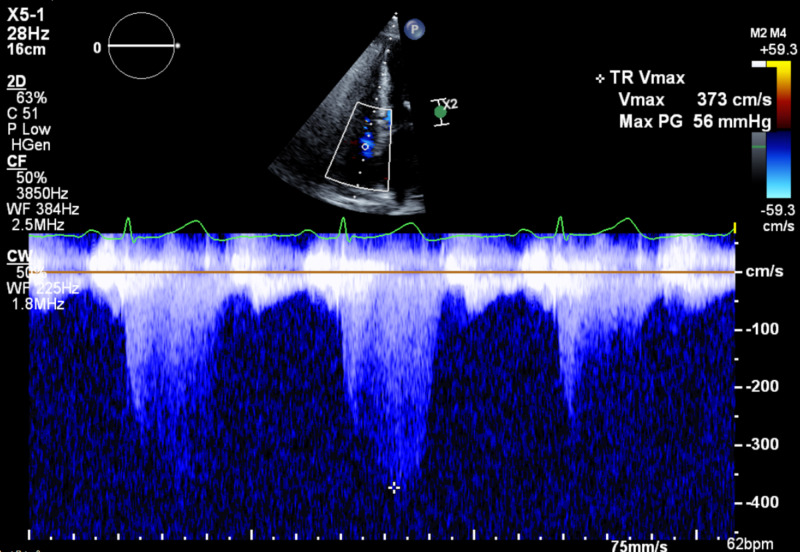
Initial TTE showing tricuspid regurgitation Vmax 373 cm/s. TTE: transthoracic echocardiogram; Vmax: velocity
